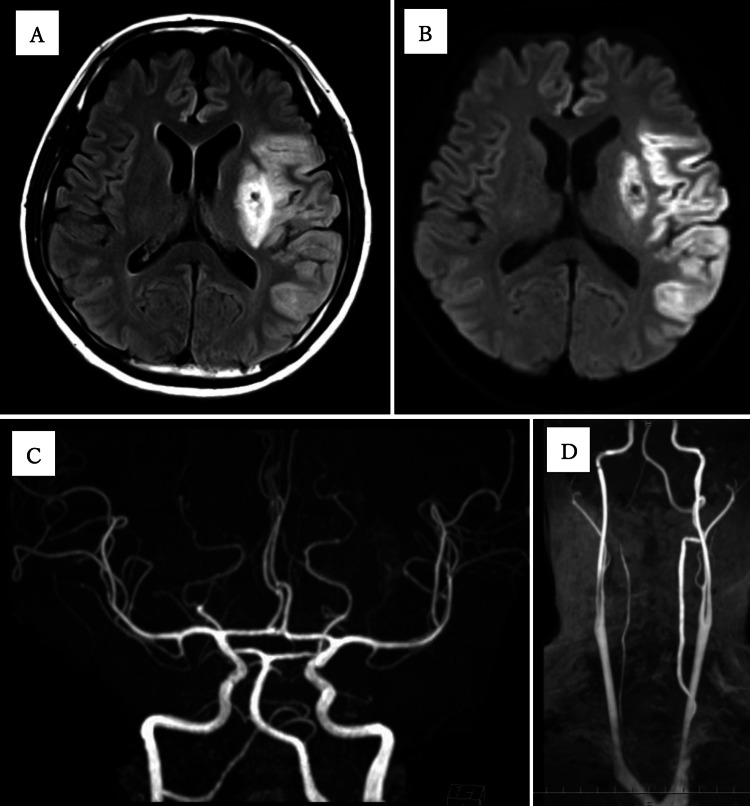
Brain MRI performed two days after onset Fluid-attenuated inversion recovery (A) and diffusion-weighted imaging (B) revealed an infarct in the left MCA region. Brain MR angiography (C) and neck MR angiography (D) revealed no stenotic lesions or obvious plaque lesions. MCA: Middle cerebral artery; MR: Magnetic resonance
